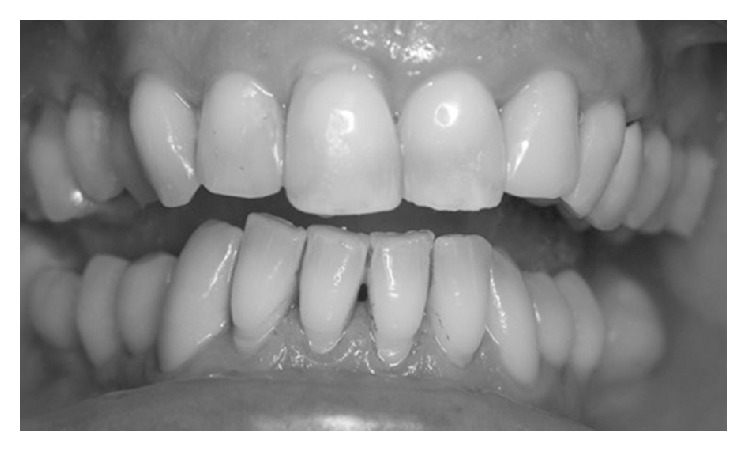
Ultrasonic debridement has been performed.
